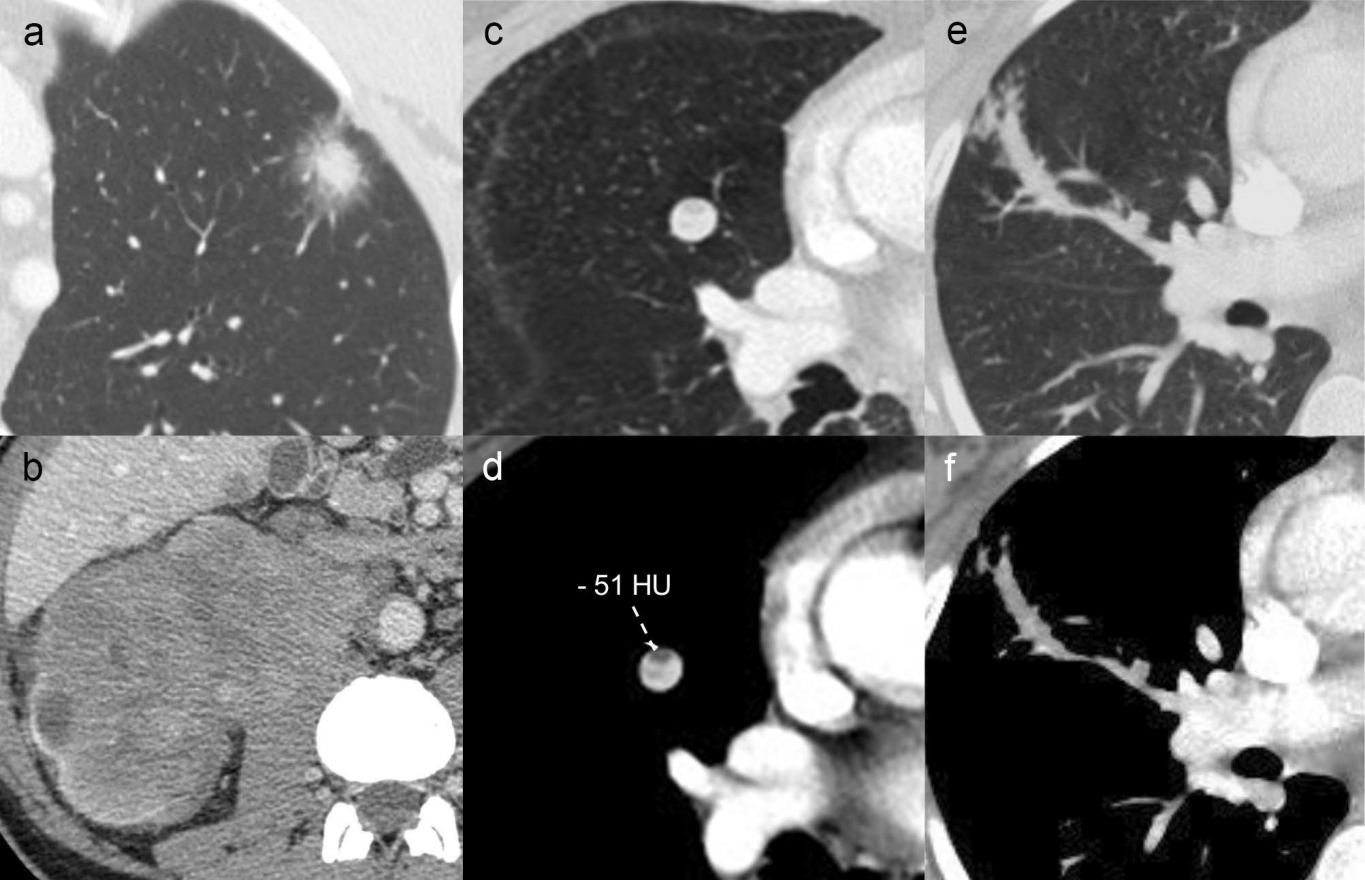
Uncommon CT features of pulmonary metastases. Metastases with less common morphological features. a, b Pulmonary metastasis in a 47 y.o. man with renal cell carcinoma. Axial computed tomography (CT) chest image a shows a pulmonary solid nodule surrounded by a peripheral ground-glass attenuation (halo sign) in the left upper lobe. Contrast-enhanced axial CT scan of the upper abdomen b depicts a large solid neoplastic mass of the right kidney, with marked heterogeneous contrast enhancement. c, d Pulmonary metastasis in a 78 y.o. man with renal cell carcinoma. Axial CT images depict a well-defined and contrast-enhancing pulmonary nodule; note the fat tissue density within the nodule in d. e, f Endoarterial tumoral embolization in a 61 y.o. man with gastric cancer. Axial CT images show vascular tree-in-bud pattern; note the irregular enlargement and occlusion of the arterial pulmonary vessels determined by intraluminal neoplastic tissue, evident until the centrilobular core
